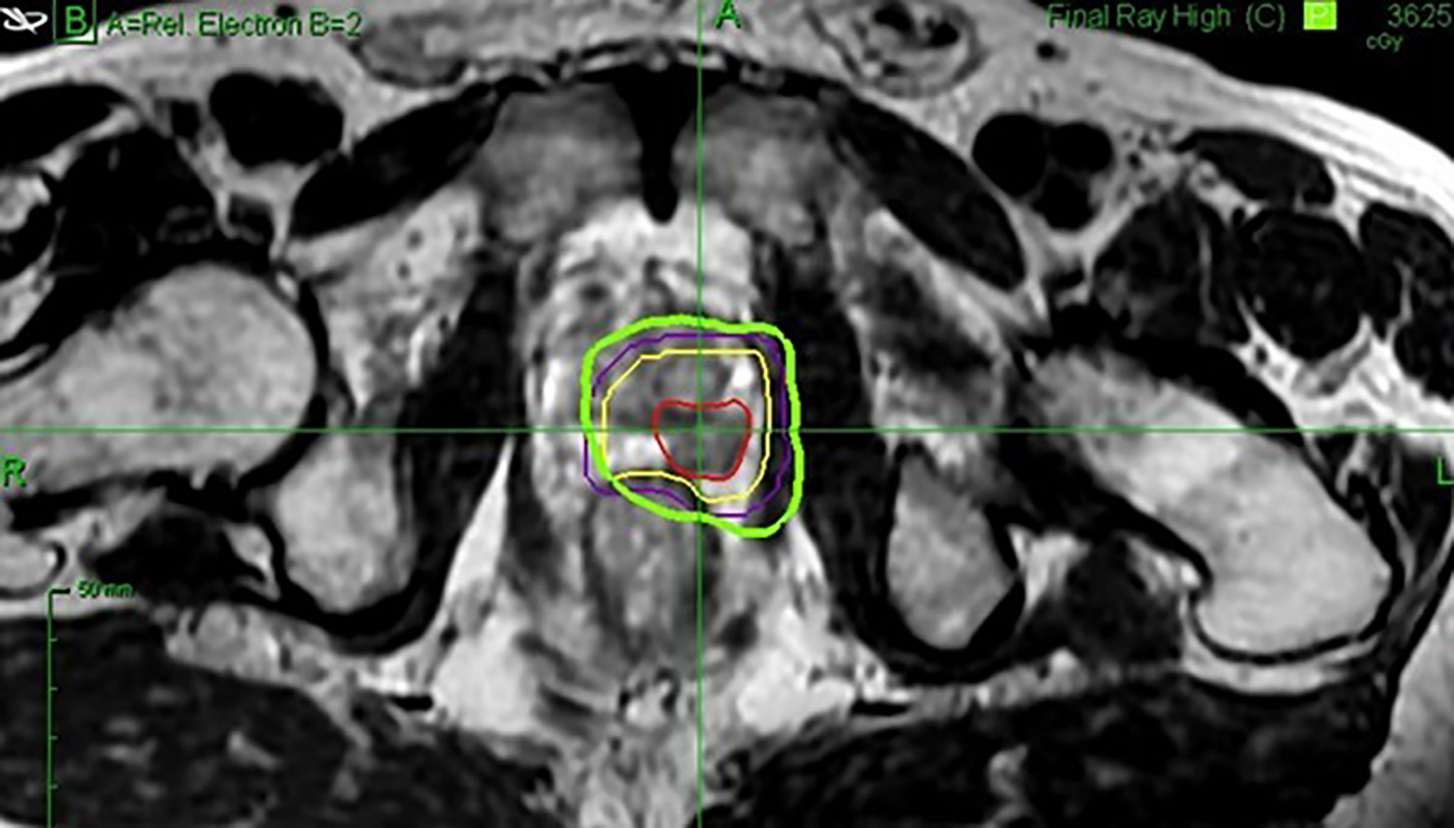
Example of a delineated focused stereotactic treatment on the MRI T2 sequence axial view. Red = gross target volume. Yellow = clinical target volume. Purple = planning target volume. Green = 36.25 Gy isodose.
